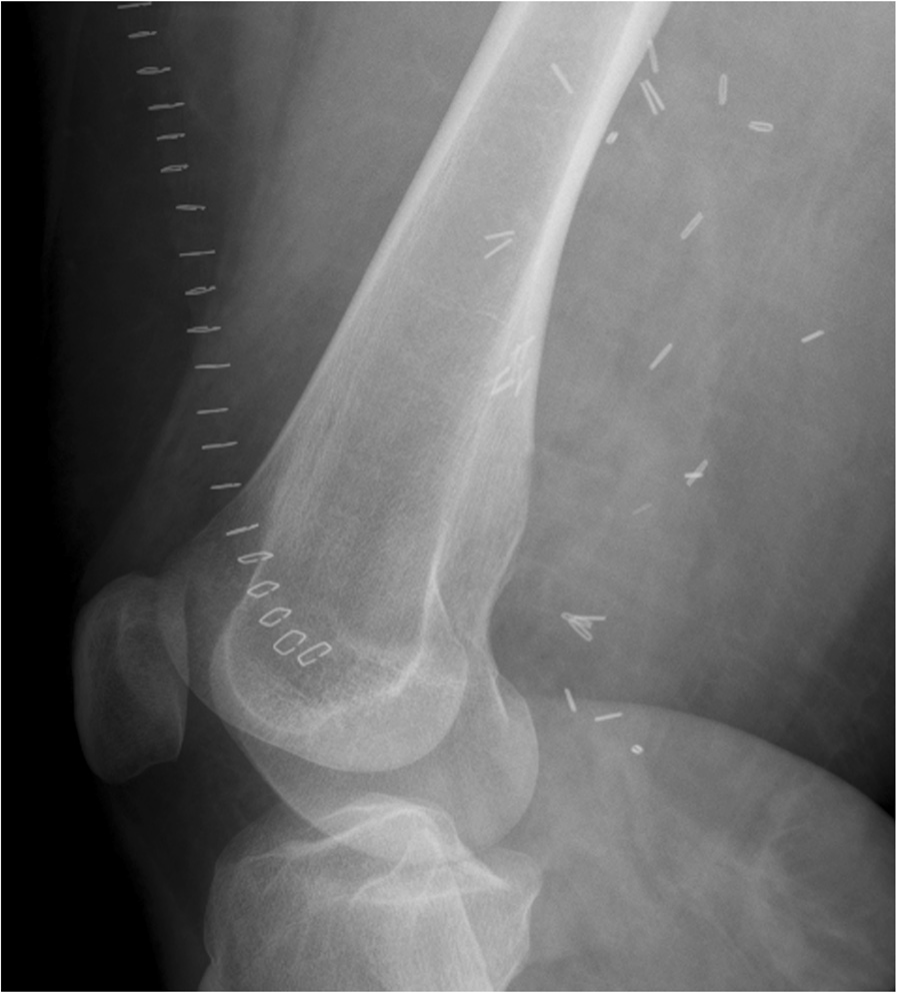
Plain x-ray after resection of the exostosis tip (lateral view).
